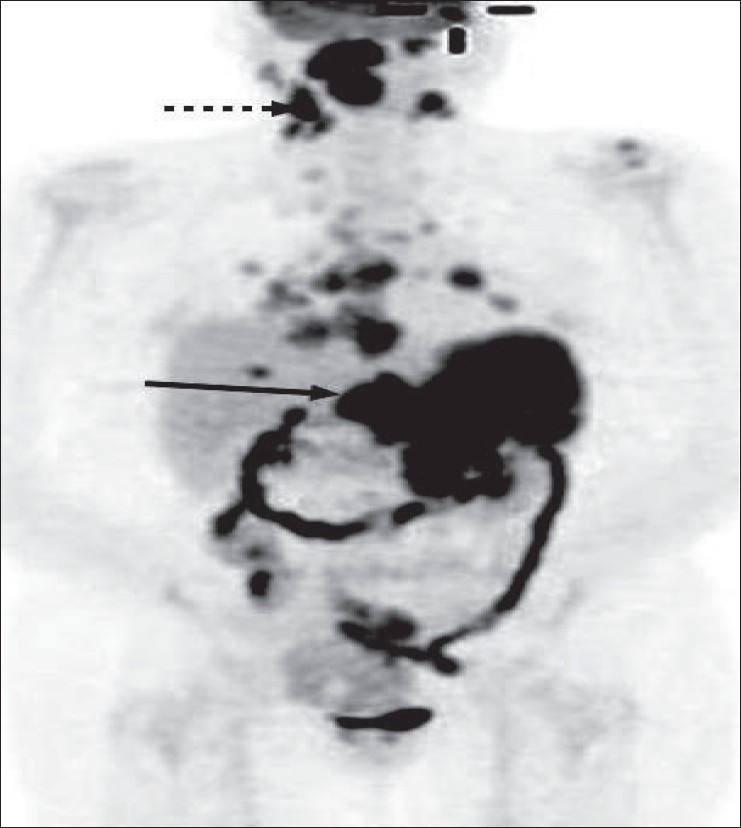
A 63-year-old lady presenting with abdominal pain was found to have a retroperitoneal mass. Biopsy revealed follicular lymphoma. MIP image from a staging PET study shows widespread involvement, the retroperitoneal mass showing intense FDG uptake (arrow). However, the neck nodes also show intense uptake (dotted arrow); and if this was known earlier, it would have presented a more accessible option for biopsy and confirmation of the diagnosis
